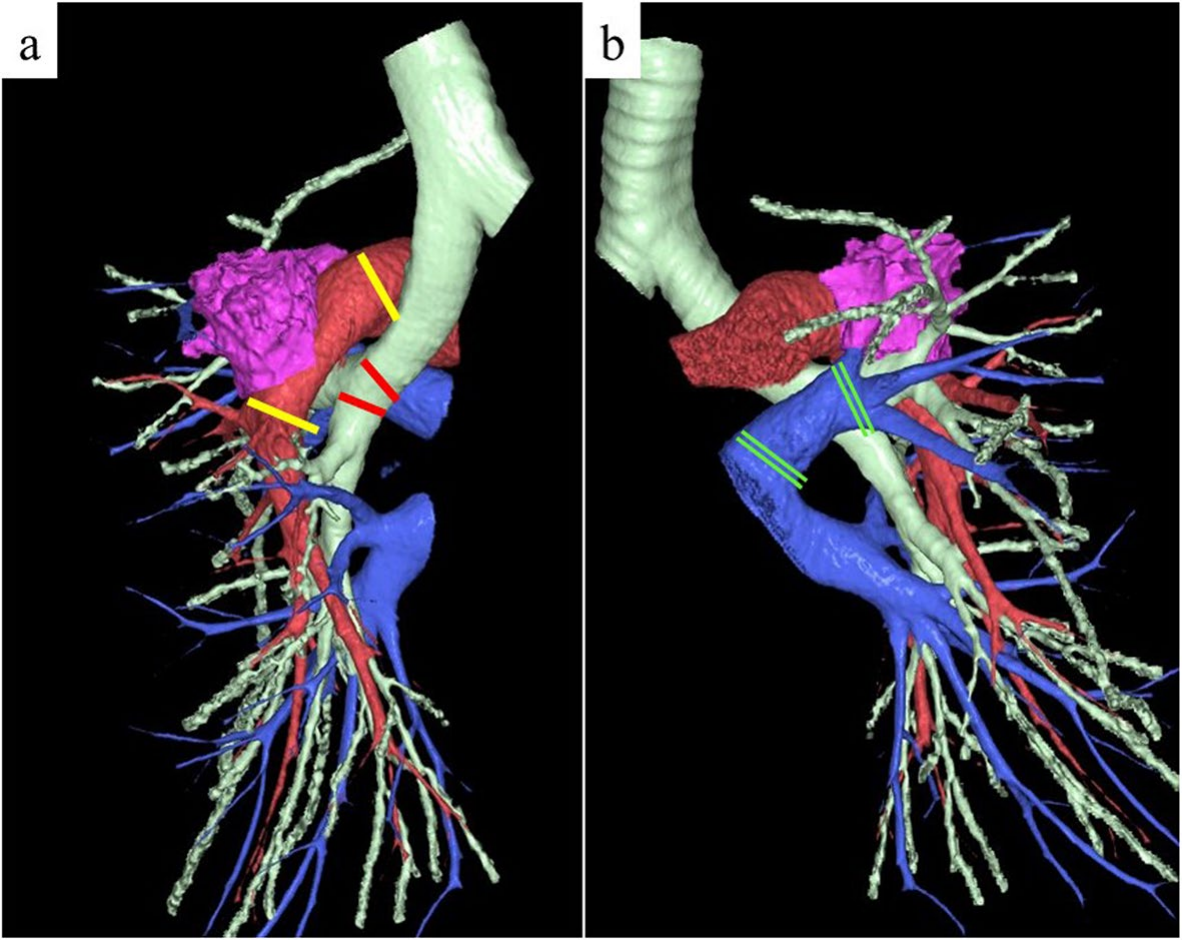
Preoperative three-dimensional computed tomography angiography of case 1. We anticipated that the length of the PA dissection would be longer than that of the bronchial dissection a and a 25–30 mm section of the left superior PV could be retrieved for an autologous conduit b
